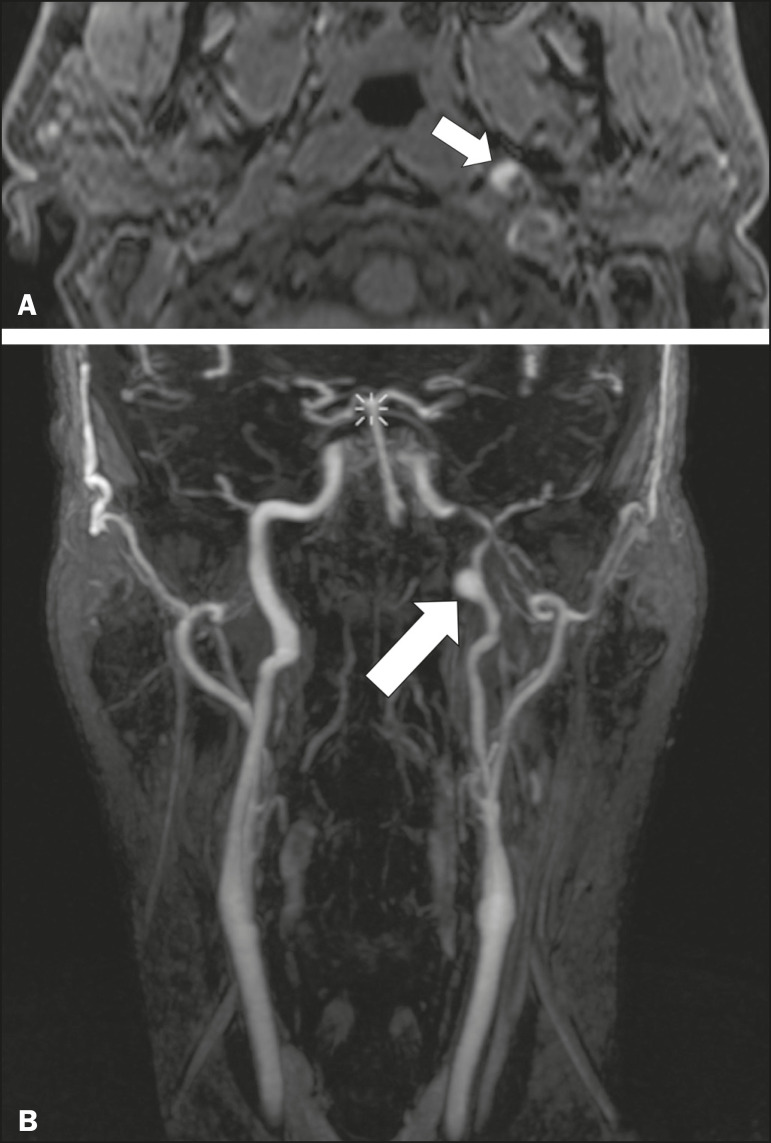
Carotid artery dissection. A 48-year-old male with sudden left cervical pain accompanied by gustatory changes. Axial T1-weighted fat-saturated MRI sequence (A) and coronal 3D time-of-flight magnetic resonance angiography (B) showing signs of recent dissection of the left internal carotid artery, depicting a crescent-shaped mural hematoma of methemoglobin, causing moderate to severe stenosis of the distal cervical segment. The accompanying focal dilation (0.7 cm) in the upper cervical segment is consistent with a pseudoaneurysm.
